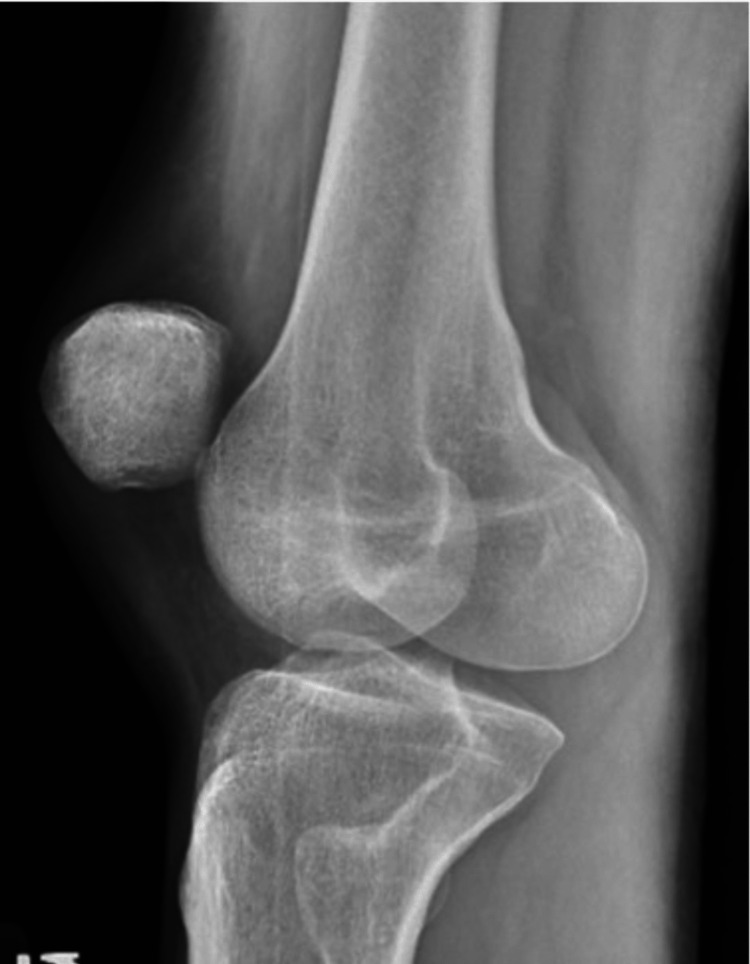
Lateral X-ray showing the patella in an unusual position
